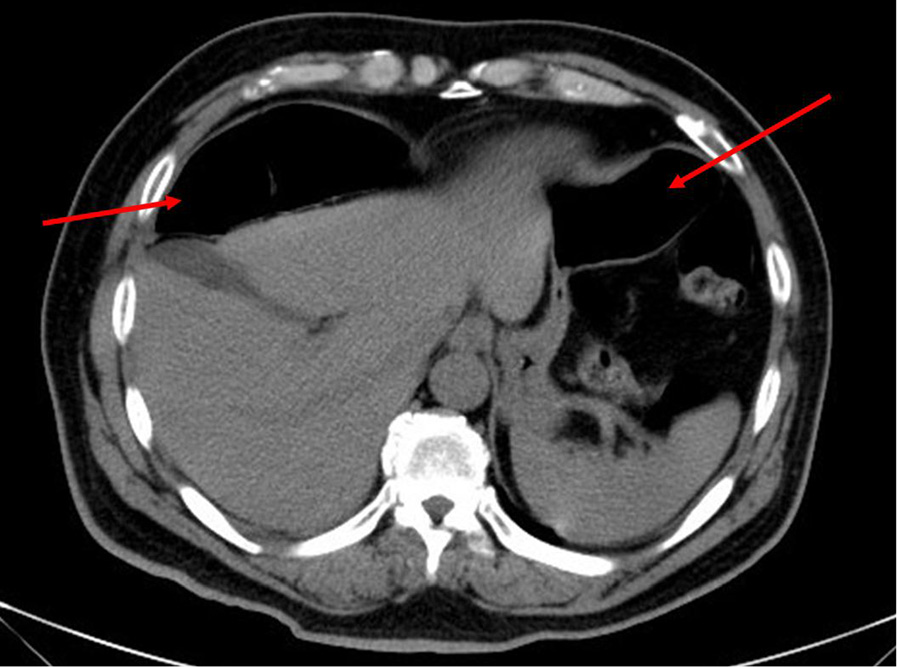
Computed tomography of the abdomen. Red arrows show the interposition of bowel loops between our patient’s liver and diaphragm.
